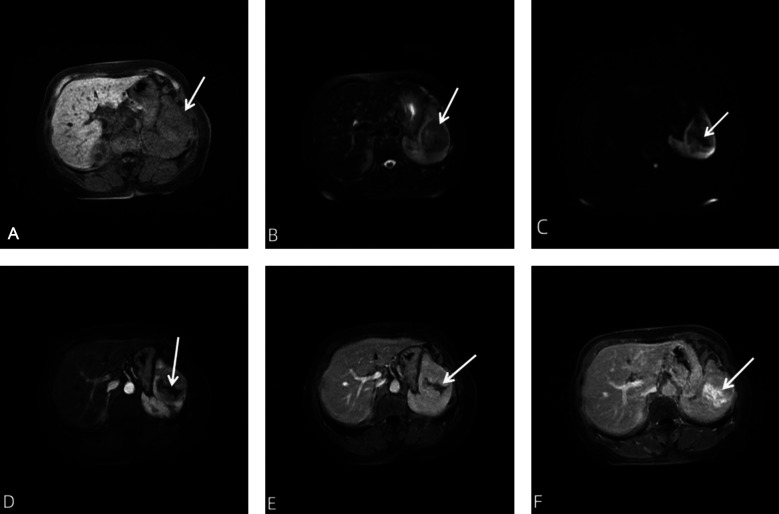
MRI of the patient's spleen. (A) Conventional spleen MRI scan showed a type of round mass in the spleen (white arrows). Most of the lesions on T1WI showed isointensity shadows, and localized slightly high signal shadows. (B) Most of the lesions on T2WI showed slightly low signal shadows, with strip-shaped high and low mixed signal shadows (white arrows). (C) In the DWI sequence, the overall signal was low, and lower signal shadow was seen inside (white arrow). (D–F) Contrast-enhanced MRI showed obvious enhancement in the arterial and venous phases of the lesion, with strip-like unenhanced and enhanced areas inside (white arrows) in delayed scan, and isointensity shadows in the remaining areas. DWI, diffusion-weighted imaging; MRI, magnetic resonance imaging; T1WI, T1-weighted imaging; T2WI, T2-weighted imaging.
